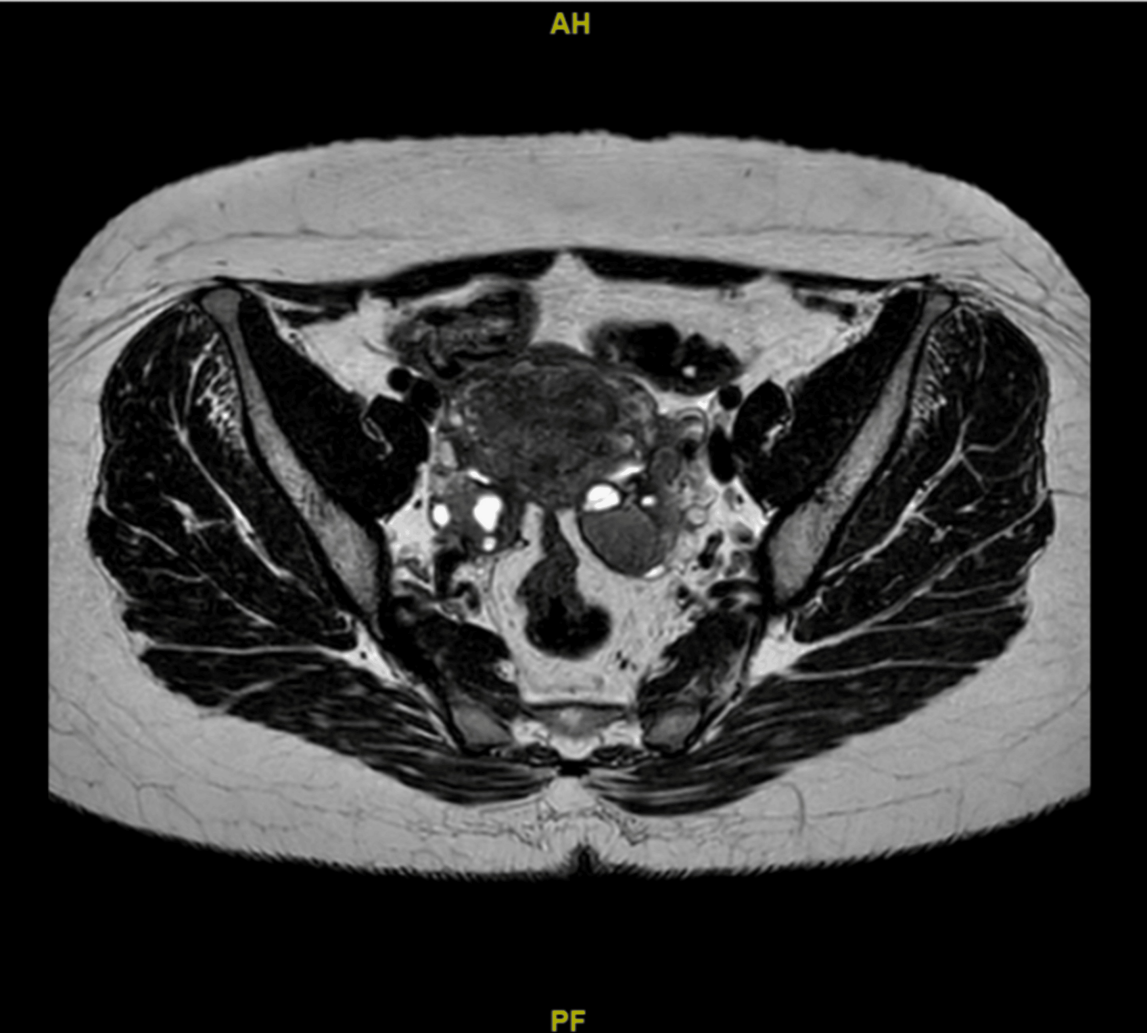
Axial magnetic resonance imaging of the pelvis after six doses of gonadotropin-releasing hormone analogue therapy, demonstrating the significant reduction of both the rectal endometrioma and the left ovarian endometrioma.
